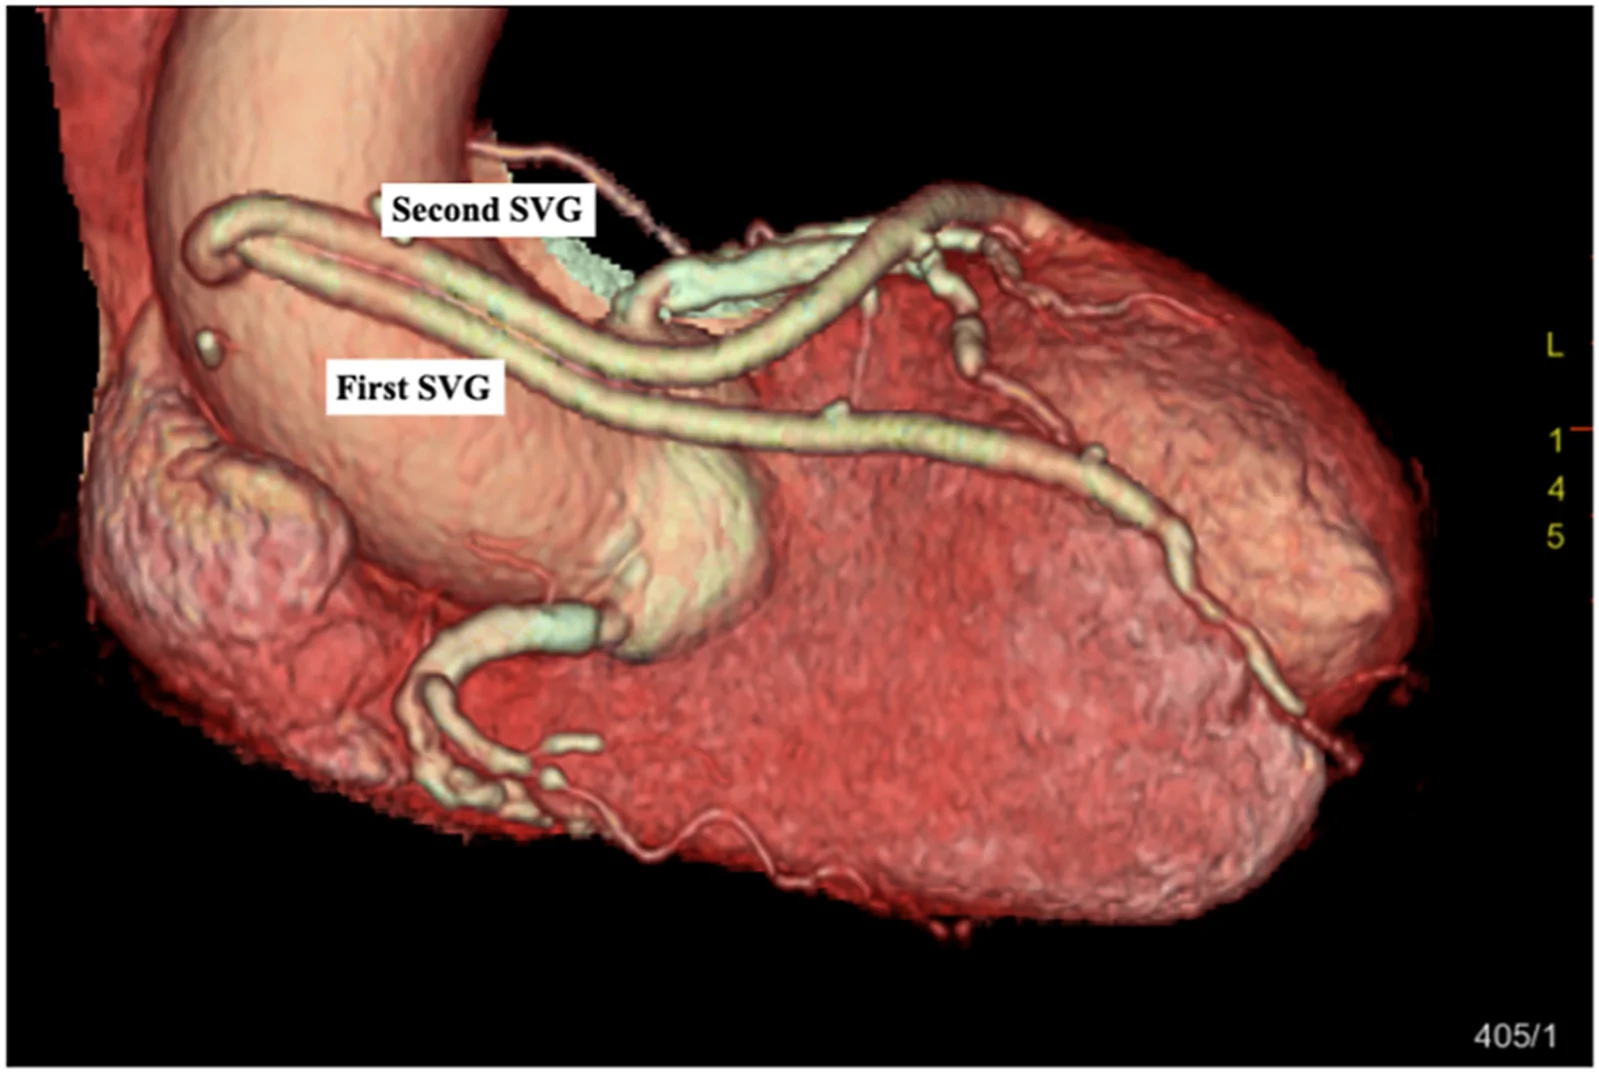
Postoperative contrast-enhanced computed tomography demonstrated patency of all bypass grafts. SVG = saphenous vein graft.
